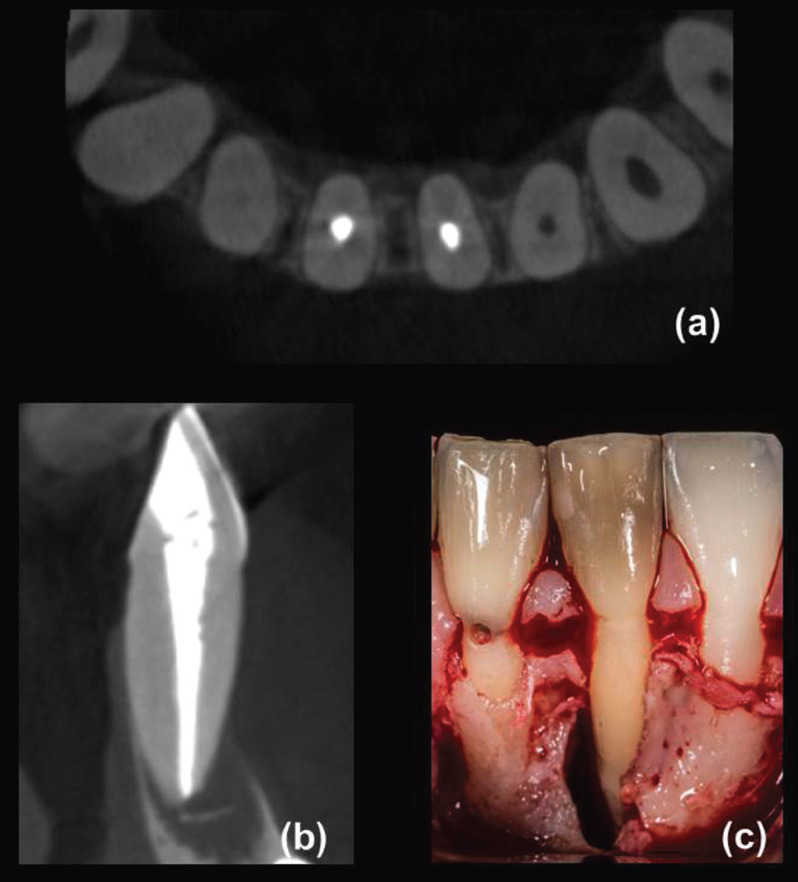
Mandibular left central incisor with persistent apical periodontitis and apicomarginal communication suggestive of VRF. (a) Axial CBCT view revealed the absence of fracture lines on the root structure. (b) Sagittal CBCT view showed a J-shaped defect extending from the periapical region to the alveolar crest. (c) Surgical approach showing a large bone defect due to apical pathosis with communication to the alveolar crest without evidence of fracture lines.
